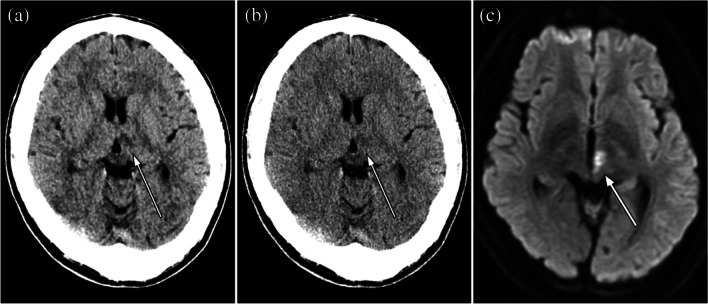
Unenhanced head CT (a, b) of a 37-year-old male patient with acute infarct in the left posterior circulation region (white arrows). The time interval from onset to CT examination was 5 h. The conspicuity of this acute infarct was rated as 5, 3, and 1 in DLR (a) and 3, 2, and 2 in Hybrid IR (b) by readers 1, 2, and 3, respectively. Window level / width is 25 / 50 Hounsifield unit for both (a) and (b). Diffusion-weighted image is also shown in (c)
